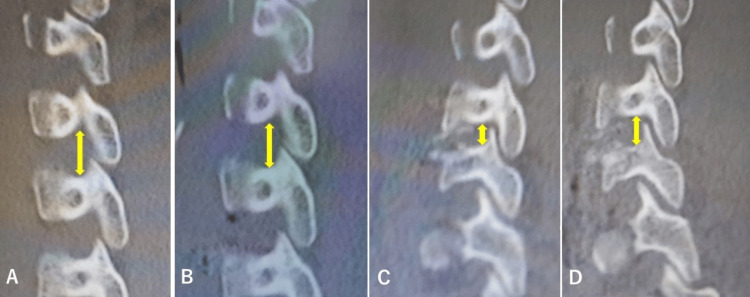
Case 2: Sagittal reconstructed CT scan. Sagittal reconstructed CT scan showing that the diameter of the left C5/6 intervertebral foramen (⇕) was 9.0 mm before the first operation (A), 9.3 mm after the first operation (B), 4.9 mm before the second operation (C), and 7.9 mm after the second operation (D).
